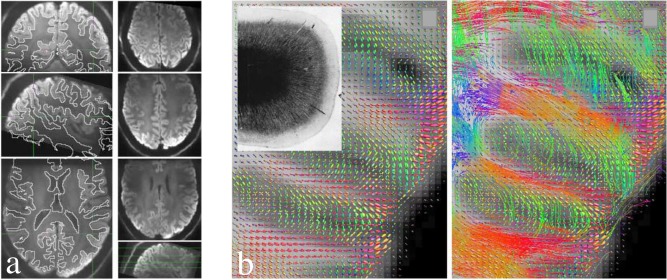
High‐resolution (0.8 mm isotropic) diffusion MRI data acquired using a combination of reduced FOV and parallel imaging method at 7T. (a) Left column: Trace‐weighted images overlaid with white/gray matter boundaries obtained from an anatomical scan, demonstrating high geometric fidelity achieved by combining reduced FOV methods and parallel imaging. Right column: axial slices at different brain regions. (b) Fiber orientation density (left) and streamline tracking (right) based on the high‐resolution data, depicting white matter fiber tracts entering the cortex. Figure reproduced with permission from Ref. 66.
